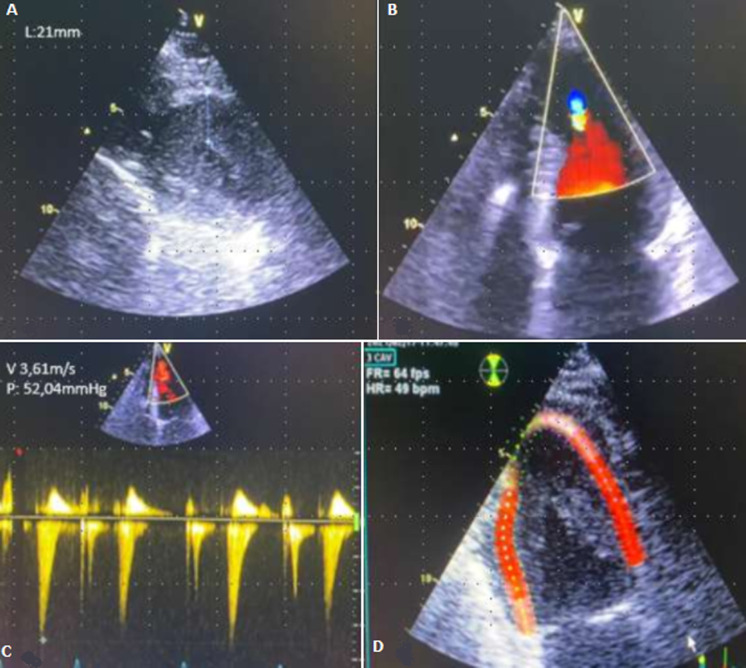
transthoracic echocardiography; A) apical hypertrophic cardiomyopathy with a wall thickness up to 21mm; B, C) left ventricular obstruction; D) altered apical strain
